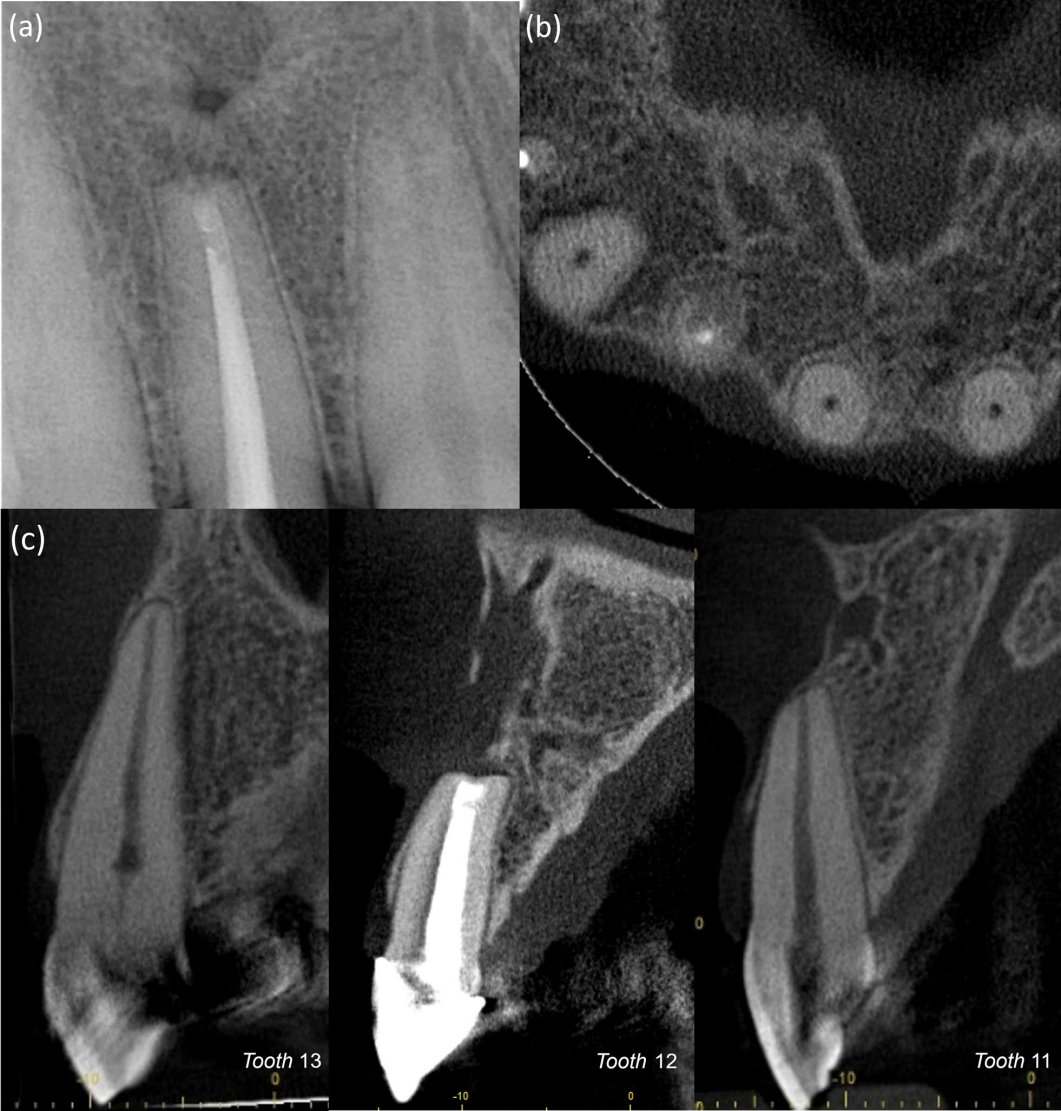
Four years after the surgery. a Periapical radiograph. b Cone-beam computed tomography images. Axial plane at the resected root level. c Sagittal plane on tooth 13, 12 and 11
